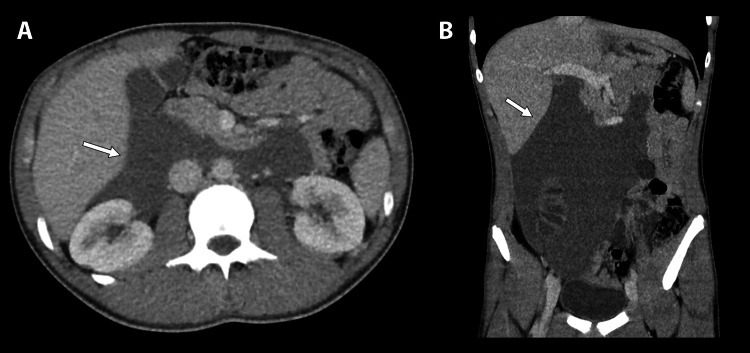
(A) Axial contrast-enhanced CT image demonstrates intermediate density free-flowing fluid in the right abdomen. The fluid displaces the pancreatic head anteriorly. (B) Coronal contrast-enhanced CT image demonstrates the extent of the free-flowing fluid throughout the abdominal cavity. Involvement includes the right paracolic gutter extending to the dome of the bladder.
